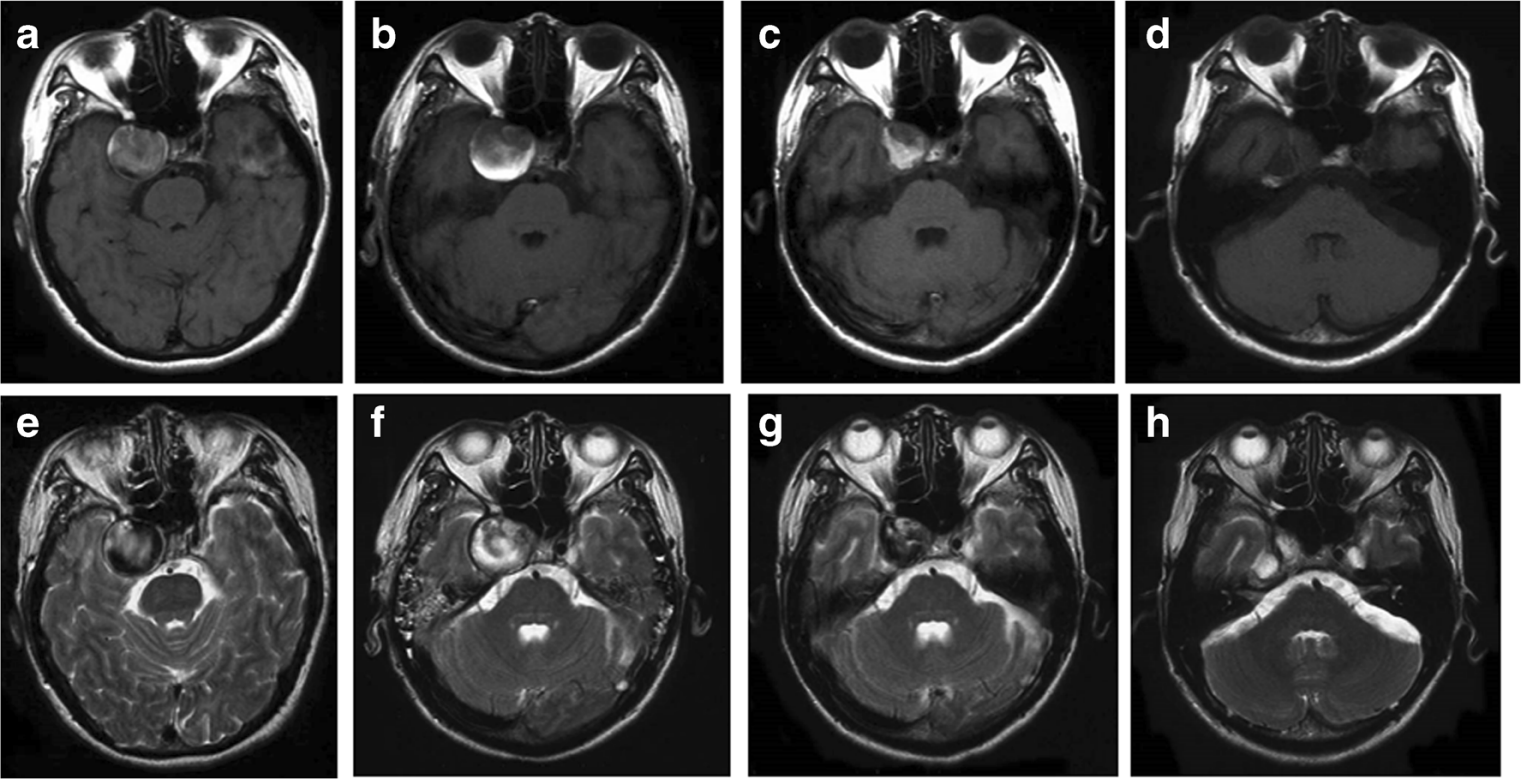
Series of MR imaging findings of a right intracavernous aneurysm before and after surgery; T1-weighted (a–d) and T2-weighted images (e–h). Preoperative MR images revealed a giant flow void and mass effect in the right cavernous portion (a, e). Notably, 1 day after surgical treatment T1-weighted (b) and T2-weighted images (f) showed high intensity inside the aneurysm, revealing the beginning of intra-aneurysmal thrombosis. Four months after treatment, the aneurysm remarkably shrank (c, g). Eighteen months after treatment the giant aneurysm almost disappeared (d, h)
